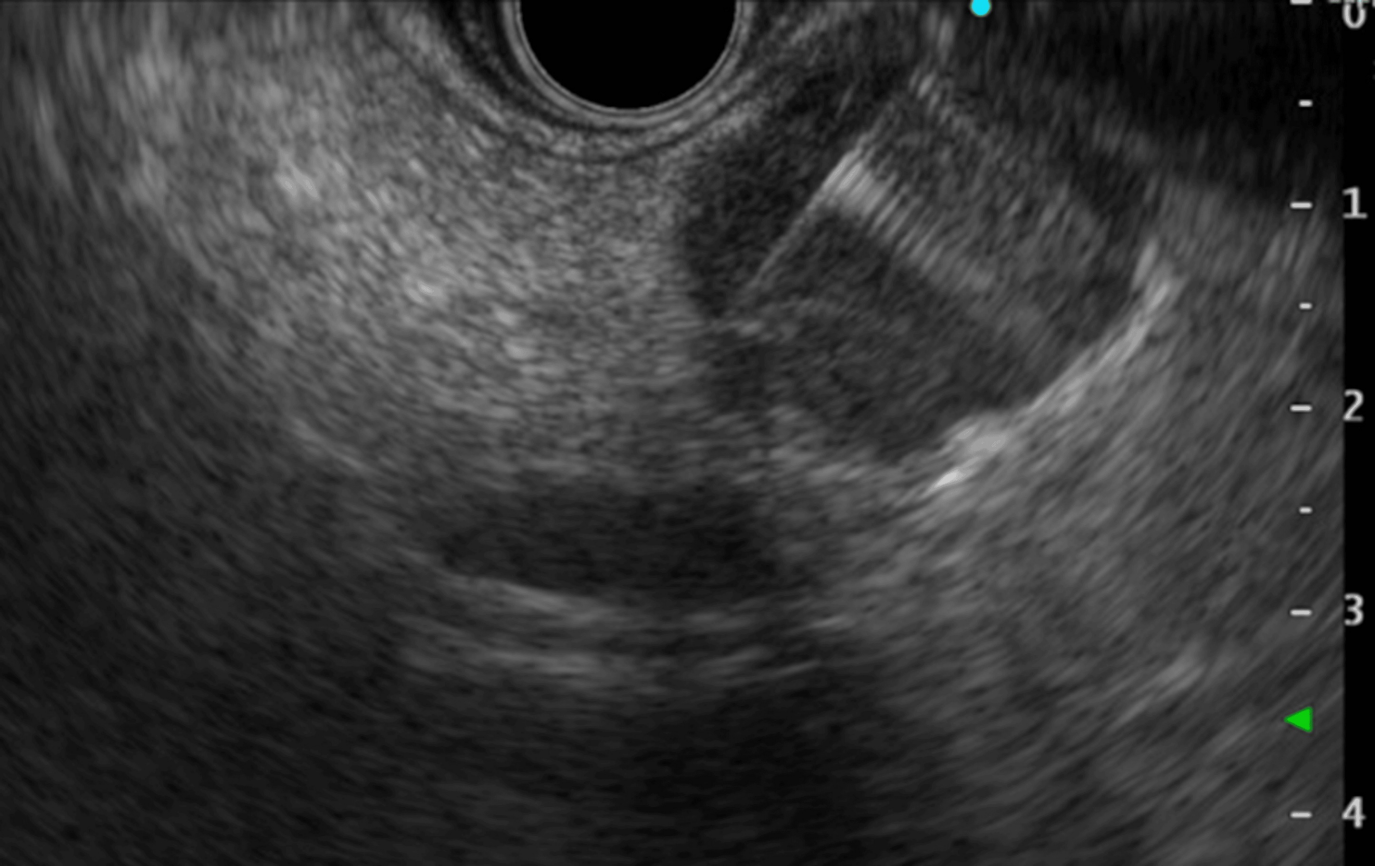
EUS image Endoscopic ultrasound (EUS) presented a low echoic, smooth surface mass on pancreatic body. EUS tissue acquisition (EUS-TA) was performed using a 22-gauge Franseen needle for two times puncture with aspiration.
